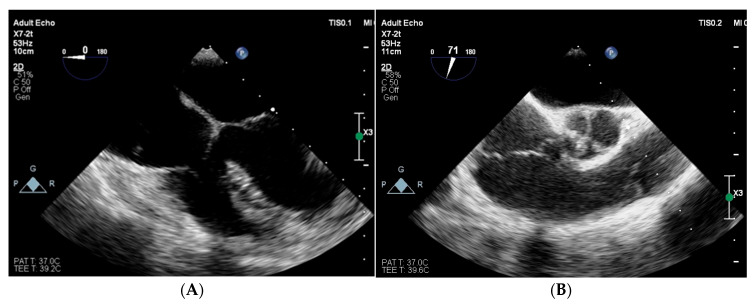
Follow-up transoesophageal echocardiography in long-axis (A) and short-axis (B) view: previously observed vegetations in the right atrium and the right ventricle have retracted and are no longer visible.
